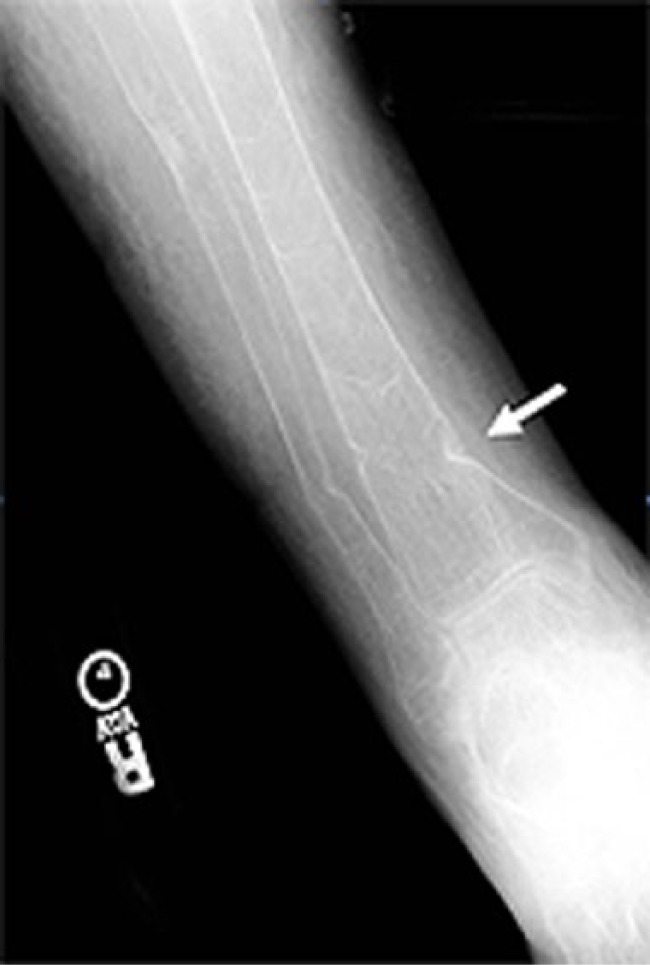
An anteroposterior radiograph of the right tibia and fibula shows striking osteopenia of both bones. Several pseudofractures (or “Looser zones”) are present, the most prominent of which is the buckle-type fracture at the distal diaphysis of the tibia (arrow).
